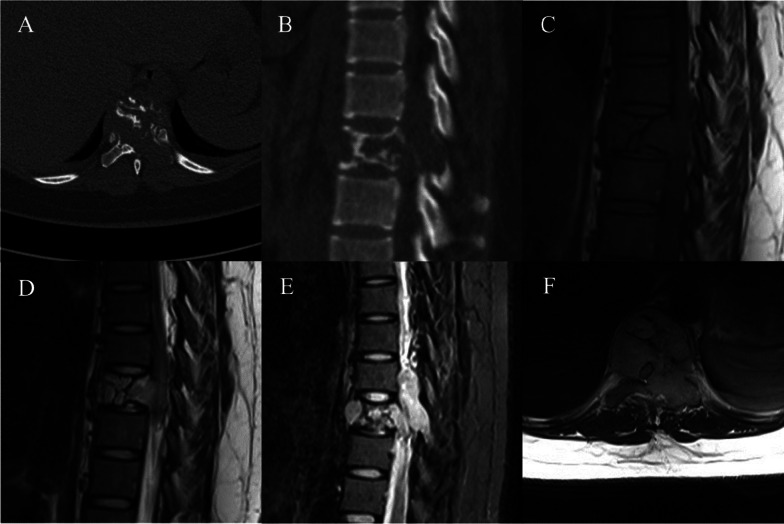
A 21-year-old woman with EHE (case 2). a Axial CT showing an ill-defined expansile osteolytic lesion of T9 with extension into the left posterior elements, containing thickened residual bone trabeculae and no sclerotic margin. b Sagittal CT showing a mild vertebral compression. c MR T1WI sagittal view showing the intermediate signal intensity (SI) of the lesion with a low-SI septum. d T2WI sagittal view showing that the tumor has high SI. e Fat suppression T2 sequence showing high SI of the lesion. f MR T2WI axial view showing a soft-tissue mass encroaching the spinal canal, which caused spinal cord compression
